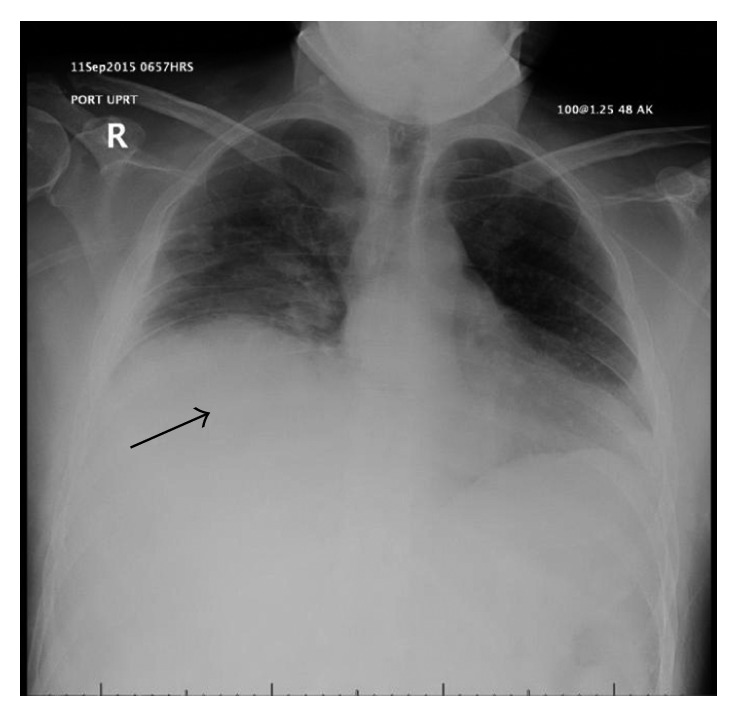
Chest X-ray shows a moderate-sized right pleural effusion (arrow), which was later confirmed to be a hemothorax.
